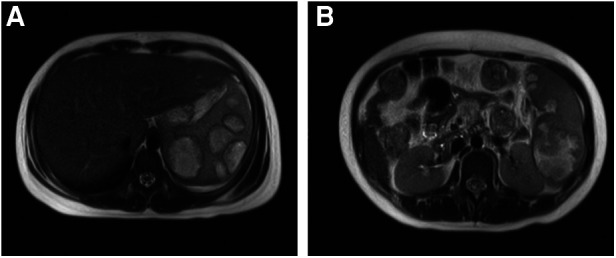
Contrast-enhanced MRI. (A): Splenomegaly with up to 7 abscesses in the same axial plane. (B): The largest abscesses.
